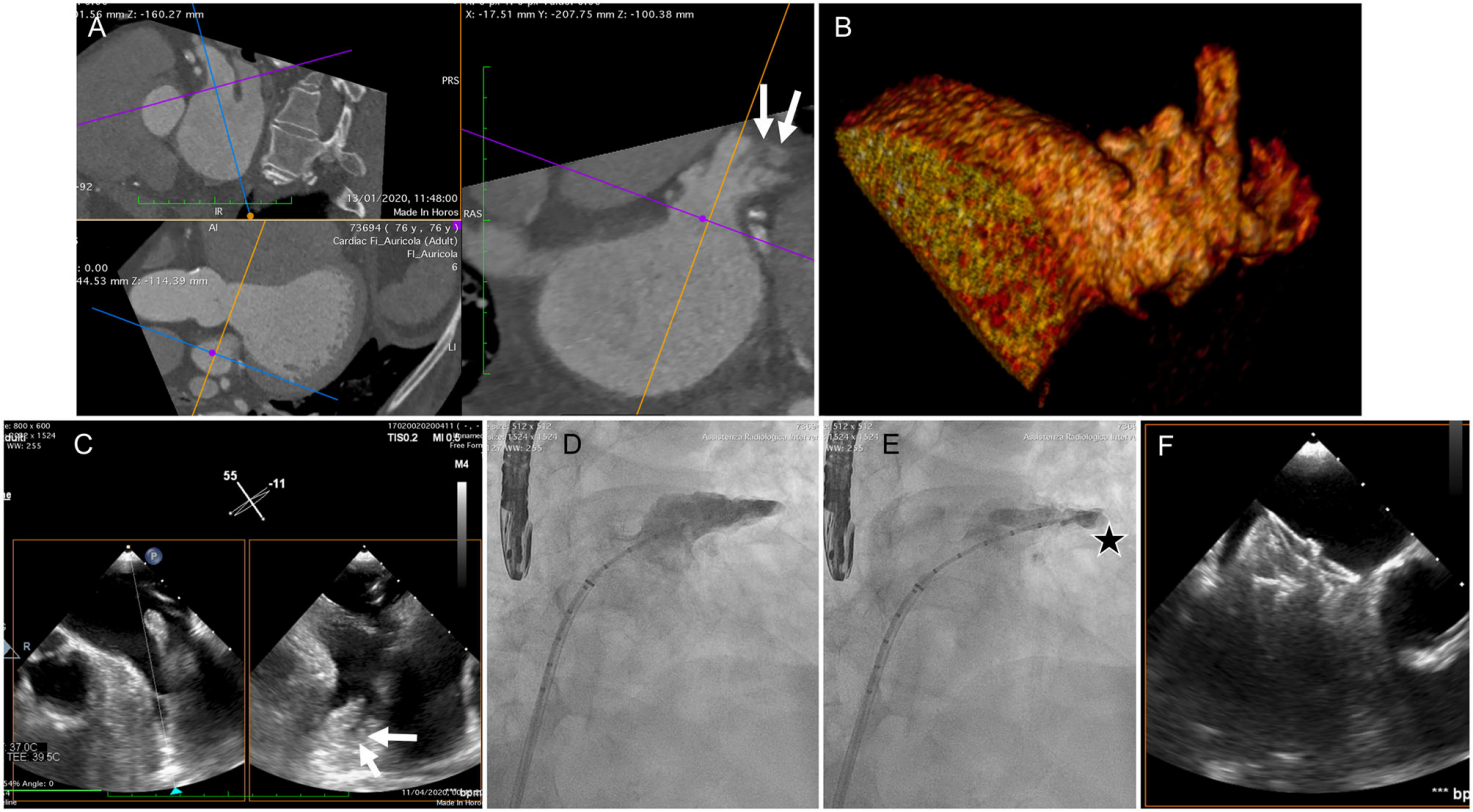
(A) CT scan of the LAA. Proximally the LAA presented a single lobe with oval-shaped ostium while distally it divided into multiple lobes, favoring blood stagnation (arrows), configuring a cactus morphology (B). (C) Transesophageal echocardiography, performed intraprocedurally revealed a “plus” image in the distal part of the appendage (arrows). (D) selective angiography revealed stagnation of dye in the distal lobe, with a slow wash out (E – star). (F) Final result after successful LAA occlusion with implantation of a 25 mm Amplatzer Amulet device (Abbott, Chicago, IL, USA).
